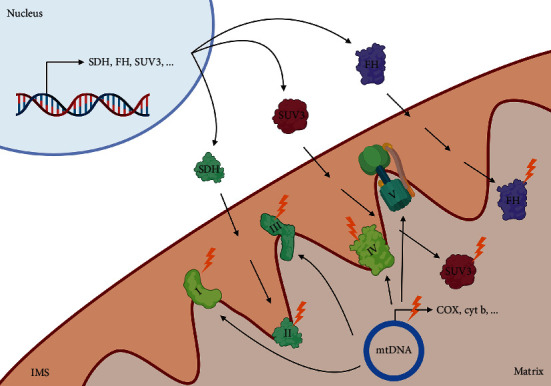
Nuclear-encoded and mitochondrial-encoded mitochondria regulatory genes are susceptible to oxidative damage. Nuclear-encoded mitochondrial genes such as SDH, which makes up complex II of the ETC, are assembled and transported into the mitochondria and susceptible to inactivation by ROS such as H_2_O_2_. In addition, FH generates energy for the cells by converting fumarate into malate in the TCA cycle, and SUV3 is a nuclear-encoded ATP-dependent DNA/RNA helicase and function as a tumor suppressor. The mtDNA encodes for subunits of the ETC and regulatory proteins that are important for the assembly and function of the ETC. The mtDNA is highly susceptible to oxidative stress-induced damage, leading to OXPHOS dysregulation. Figure created with biorender.com.
